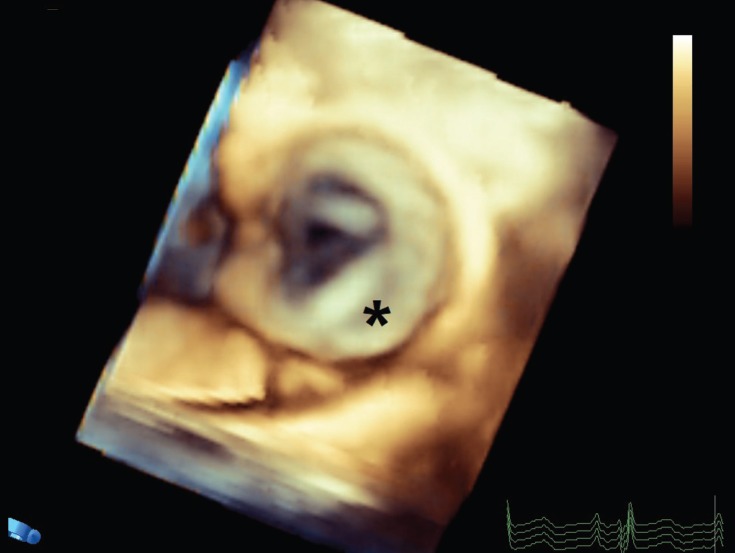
En face view of the pulmonary valve in 3D transesophageal echocardiography, showing that the tip of 1 pulmonary cusp is attached to the sinotubular junction (*).
